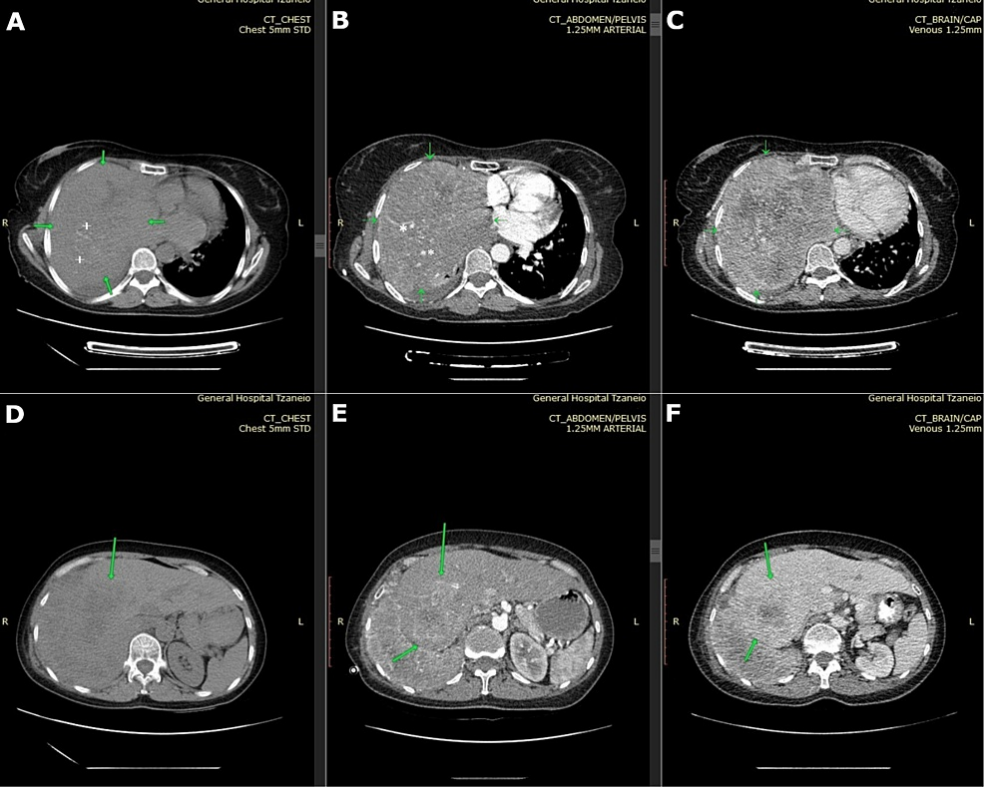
CT findings 3A-3C: Axial chest CT (A is non-contrast, B is arterial phase, and C is portal venous phase) at the level of the heart. A large heterogenous mass with central amorphous calcifications (A, crosses) and neovascularity (B, asterisks) is occupying the right hemithorax. The arrows show the mass margins. 3D-3F: Axial abdominal CT (same phases as above). The heterogeneous mass is shown with arrows at segment 8 of the liver. It has similar imaging findings to the lung mass.
